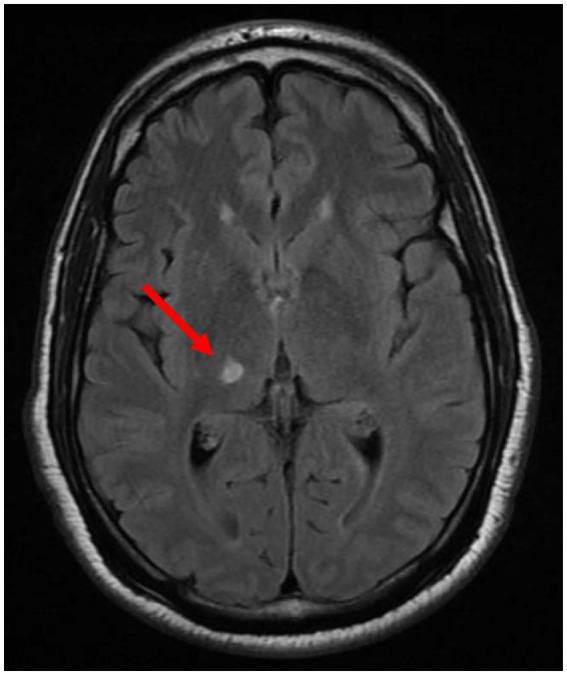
MRI January 2022 showing right thalamic lacunar infarction.
